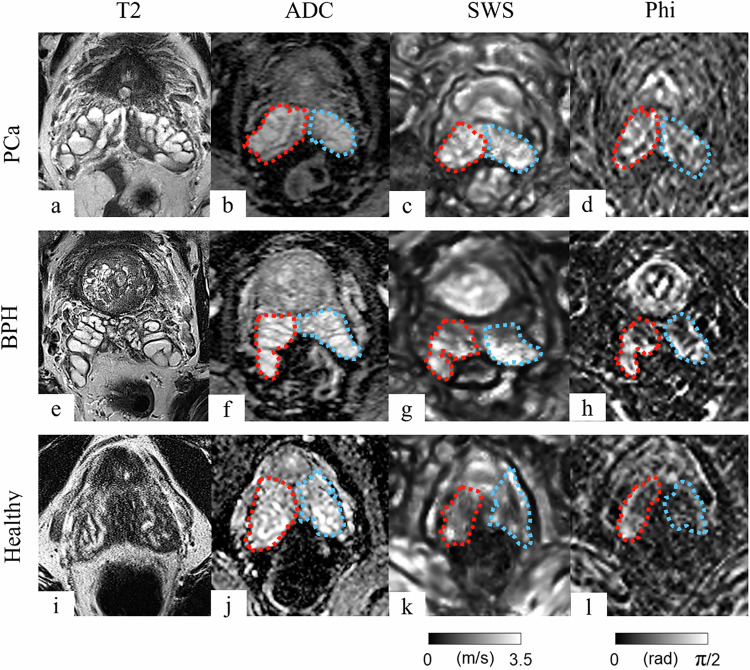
Representative multiparametric MRI and MR elastography sequences. The first row shows images from a patient with prostate cancer (PCa) (a–d), the second row from a patient with benign prostatic hyperplasia (BPH) (e–h), and the third row from a healthy volunteer (i–l). The right seminal vesicle is encircled in red, and the left seminal vesicle in blue
